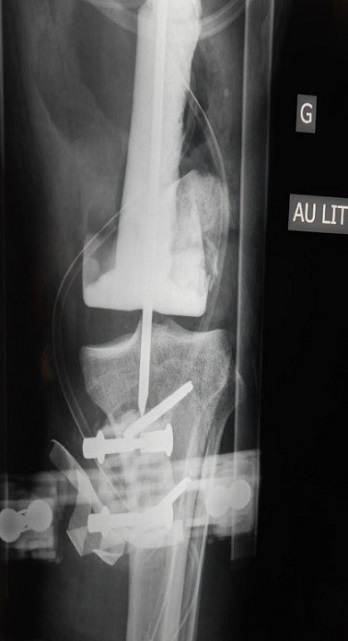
Anteroposterior radiograph of the left knee showing femoral spacer with external fixator
